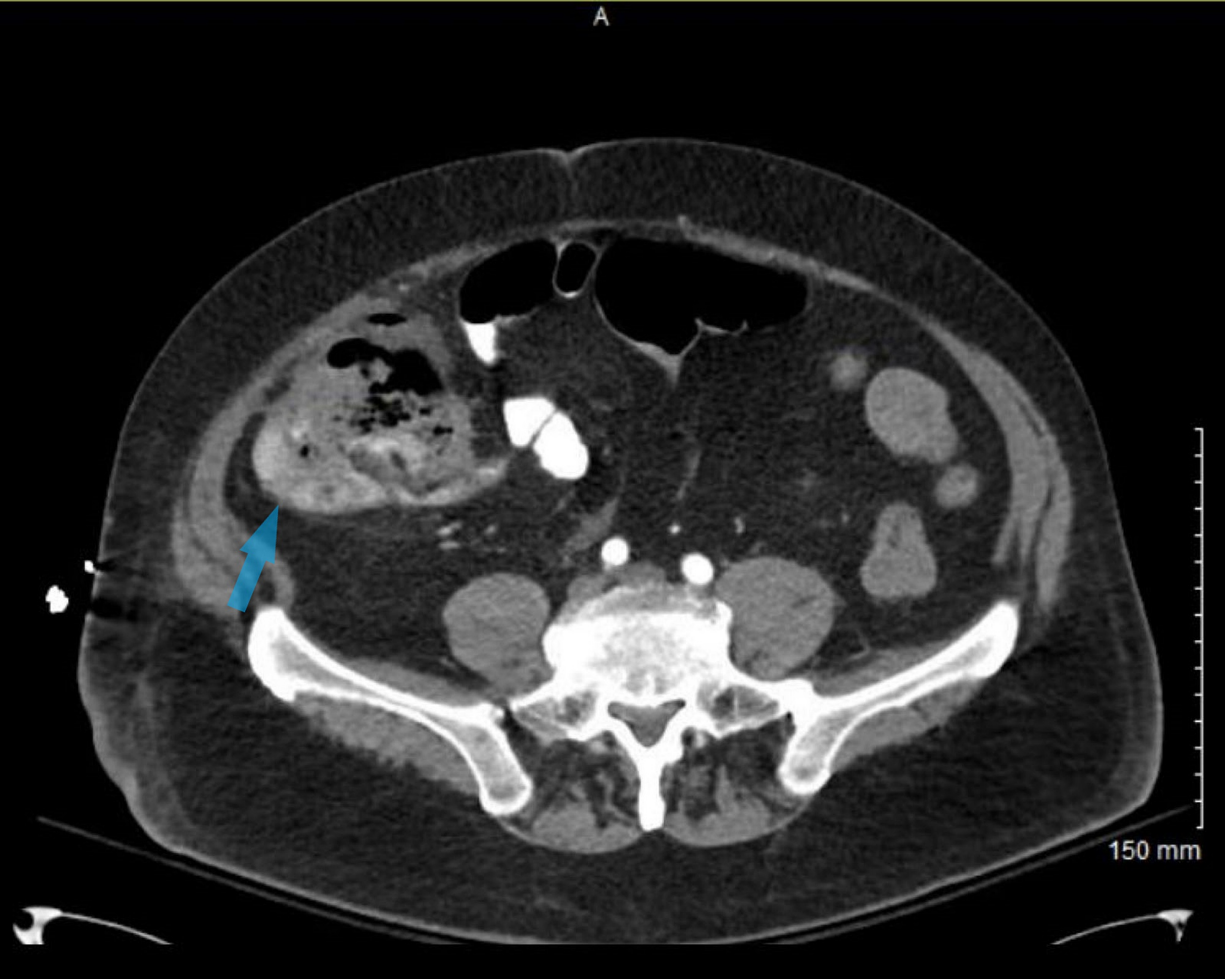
Axial view of a CT of the abdomen and pelvis with oral and intravenous contrast An 8-cm cecal mass (Transparent blue arrow) with loss of the fat plane between the cecal mass and the anterior lateral right abdominal wall with possible invasion of the wall.
